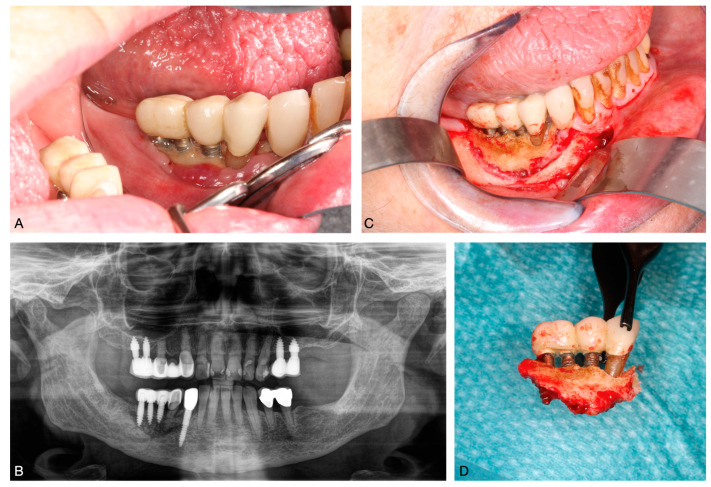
BRONJ of the lower jaw following implant placement. (A): exposed bone in the oral cavity; (B): panoramic radiograph showing a bone sequester; (C): intraoperative exposure of the sequester; (D): removed bone sequester.
